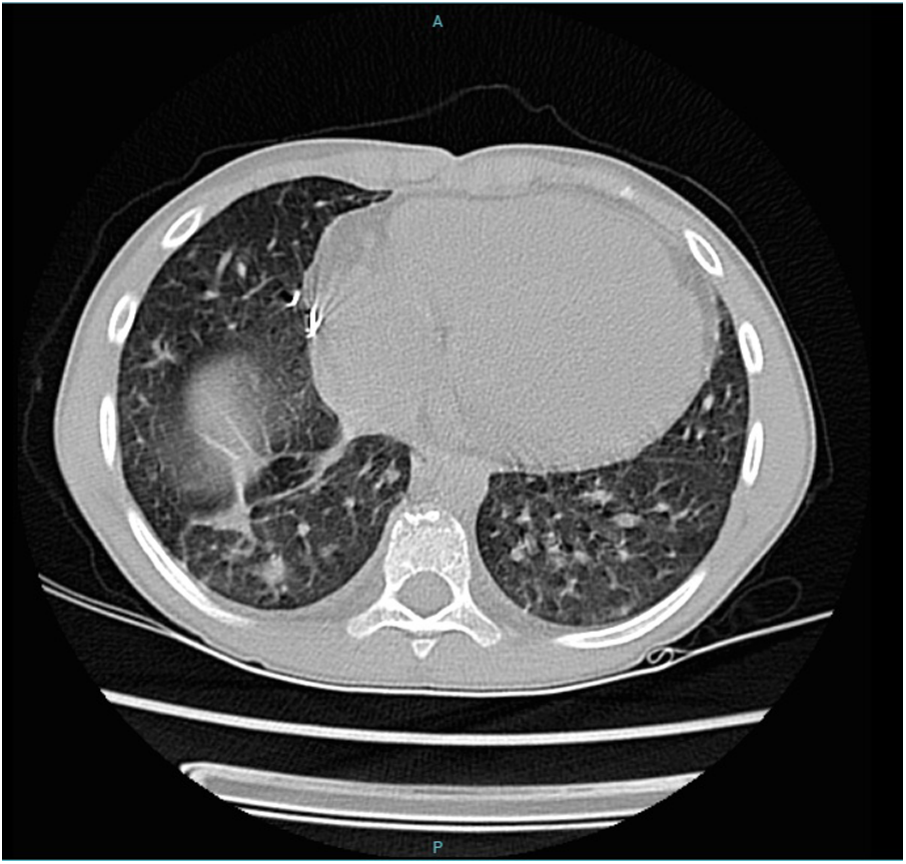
CT scan showing vegetation in the RV-PA conduit.
